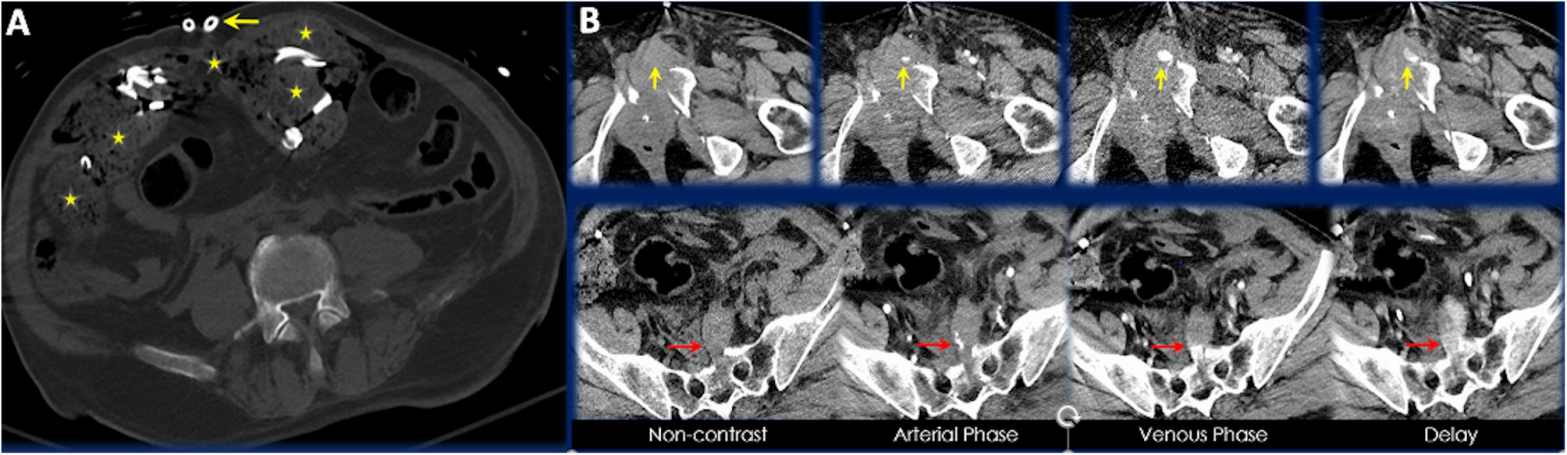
(A) Axial CT image of a 70-year-old male presenting as a trauma alert red following motorcycle accident. Placement of surgical drains, retained laparotomy sponges, and preperitoneal hematoma are visible in this axial slice. (B) Axial CT images highlighting areas of contrast extravasation in different CT phases. Yellow arrows point to extravasation anterior to an acute comminuted left superior pubic ramus fracture and red arrows point to extravasation anterior to an acute comminuted sacral fracture.
